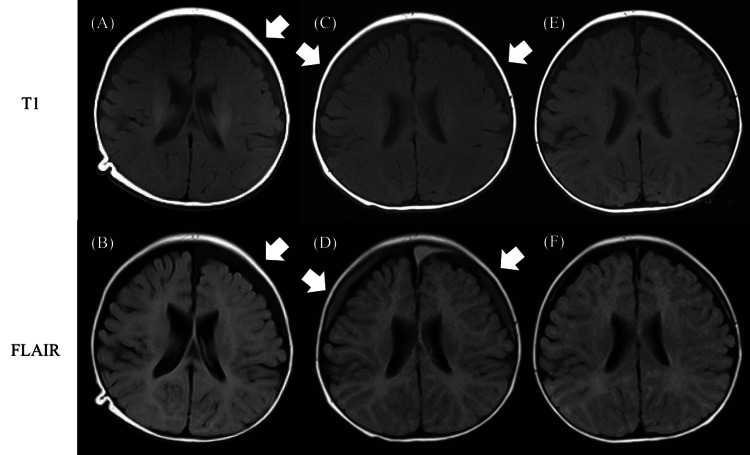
Brain magnetic resonance imaging of the patient (A) and (B) day 17 after admission. Subdural effusion (arrow) was observed in the left frontal lobe. (C) and (D) day 51 showed subdural effusion expanded bilaterally. (E) and (F) day 150 showed that the subdural effusion had disappeared.
